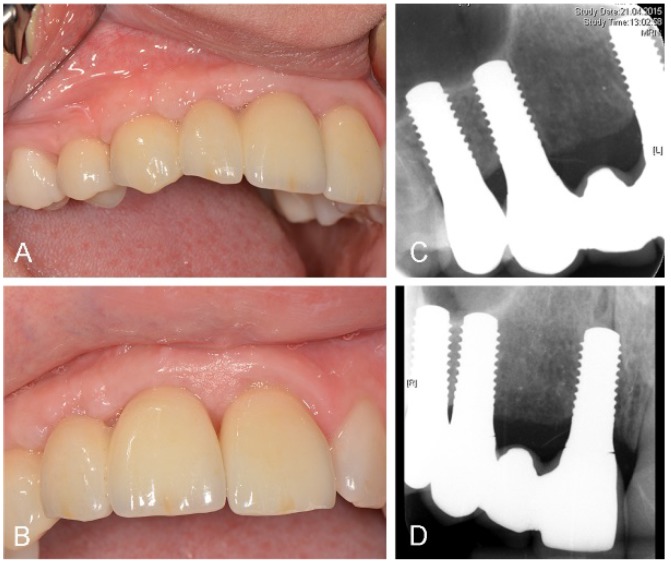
Clinical (A,B) and radiographic (C,D) investigation at 20 months after implant placement. FDP cemented on implants in region 11 and 13, single crown cemented on implant in region 14. Prosthetic reconstruction: Dr. Thomas Borer.
